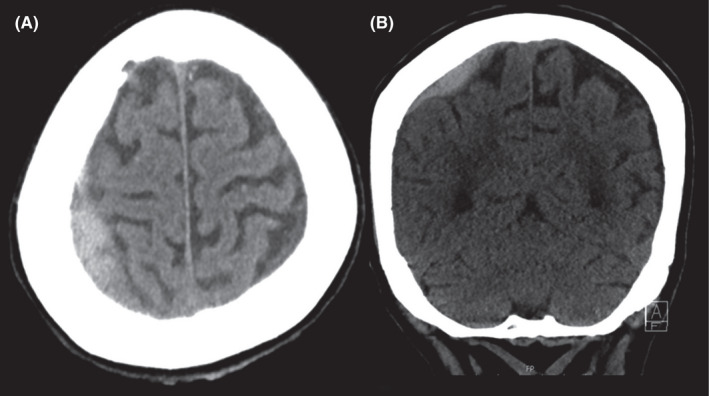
In the non‐enhanced CT scan, a right‐sided acute small (max. 8 mm) subdural hematoma, and a left‐sided chronic small (max. 3 mm) subdural hematoma were seen on the axial (A) and coronal (B) CT slices
